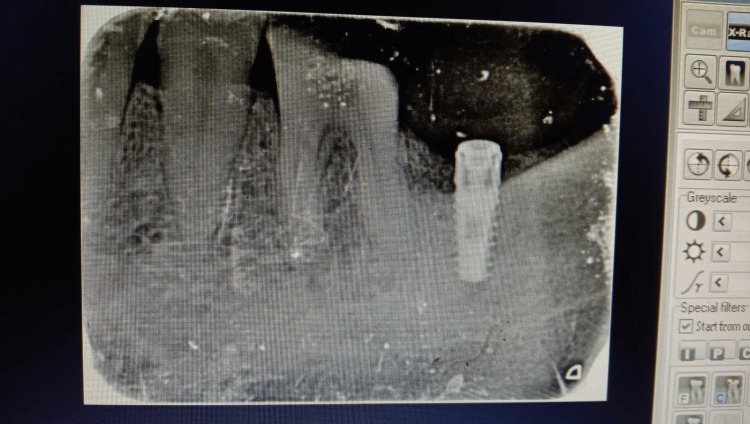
Radiographic postoperative view of implant position
